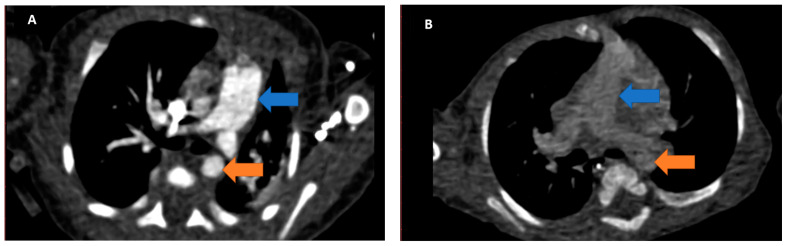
Axial CT angiography reconstruction. Blue arrow: main pulmonary artery. Orange arrow: descending aorta. (A) Excellent quality of image. The vascular structures are well-delineated due to good contrast opacification; (B) Suboptimal image quality. The vessels are difficult to delineate and reporting is impaired.
